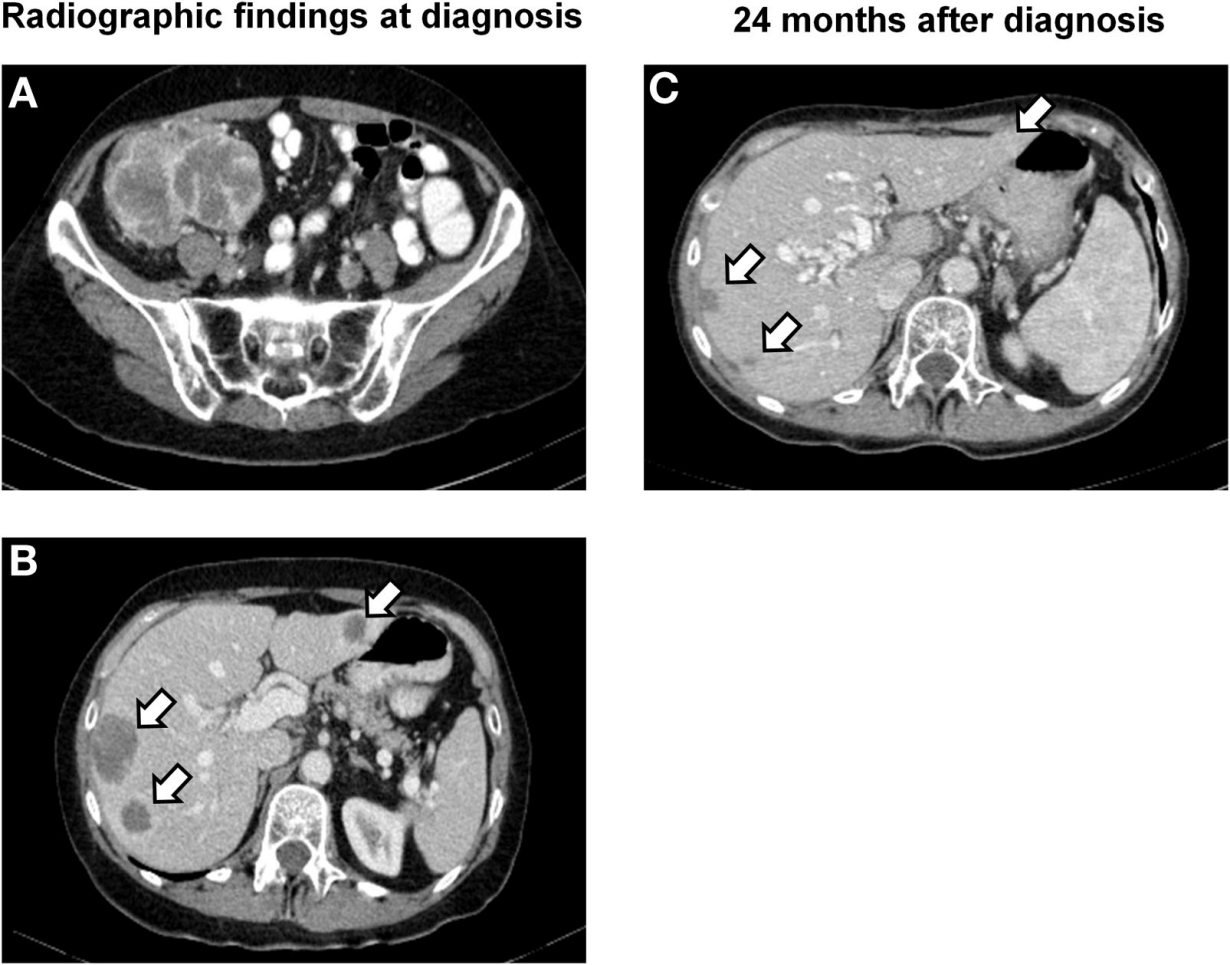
Radiographic findings. (A) CT image of the lower abdomen showing a poorly delineated, partially necrotic tumor in the ileocecal region with a maximum diameter of 9.6 cm. (B) CT scan of the upper abdomen demonstrating hepatic metastases in segments II, VII, and VIII with a maximum diameter of 2.6 cm (segment VI not visible in this image plane). (C) Follow-up examination 24 months later showing subtotal regression of the metastases in segments VII and VIII and total regression of the lesion in segment II.
